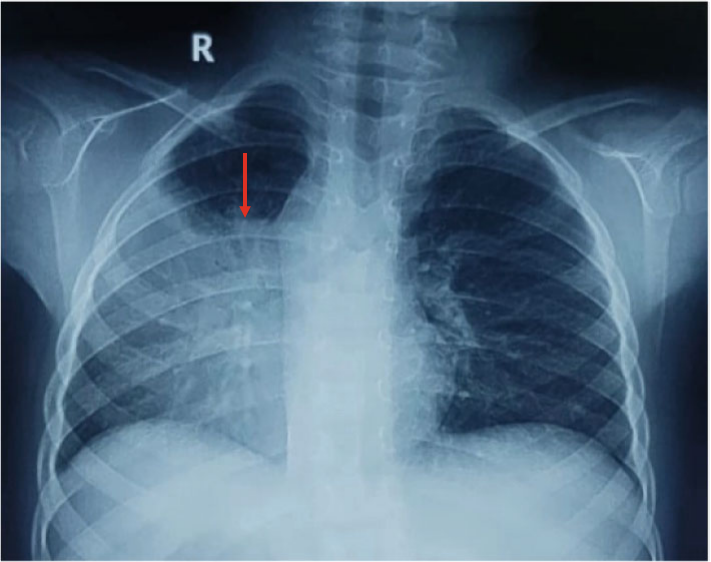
Chest X-ray showed right side mid and lower zone consolidation in nondiabetic patients.
